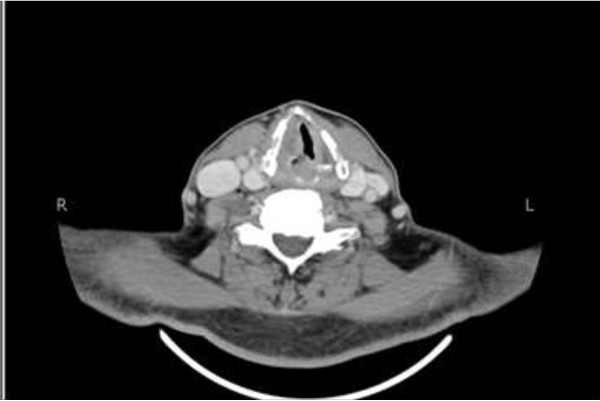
CT scan of the neck showing a hyper dense mass lesion involving right aryepiglottic fold extending into right vocal cord.
